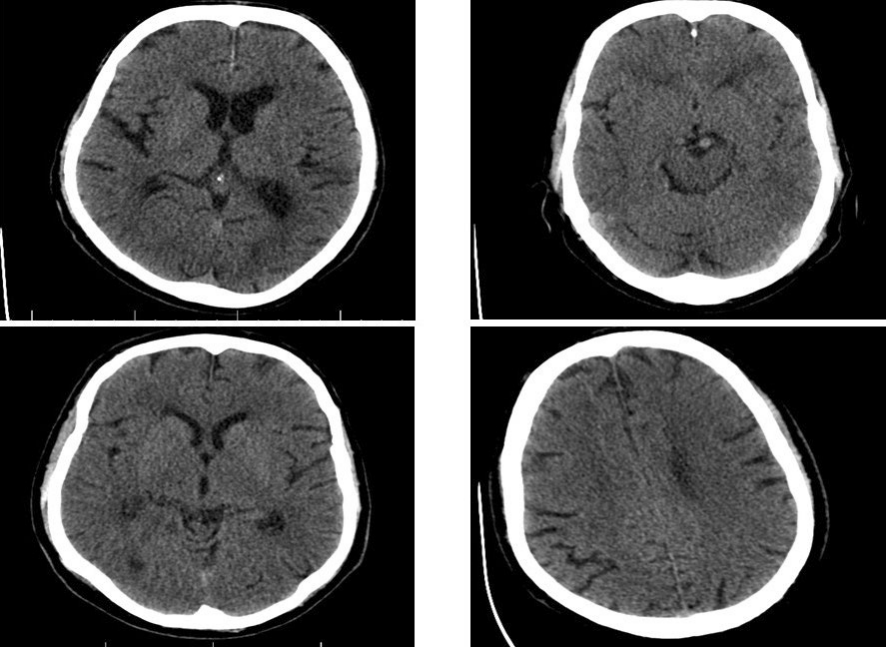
CT-scan of a number of control individuals.
